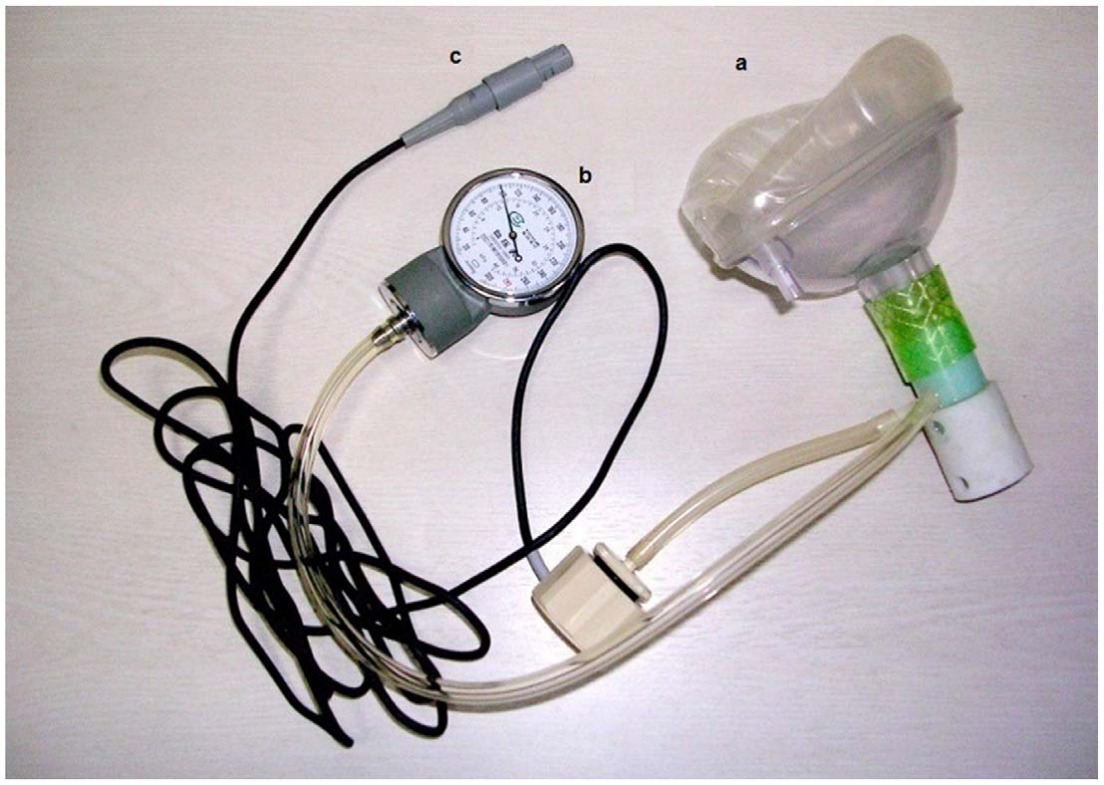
The pressure measurement device used in this study. The mask (a) with related tube was connected to the watch-shaped manometer (b), which was detached from an aneroid sphygmomanometer (XB-11 aneroid sphygmomanometer, Shanghai Medical Equipment Factory, Shanghai, China), and the needle of the manometer was set at 100 mmHg as the zero point. The pulse transducer (c) with wire and plug could be attached to the ultrasonographic system to display the action of the Valsalva maneuver.
